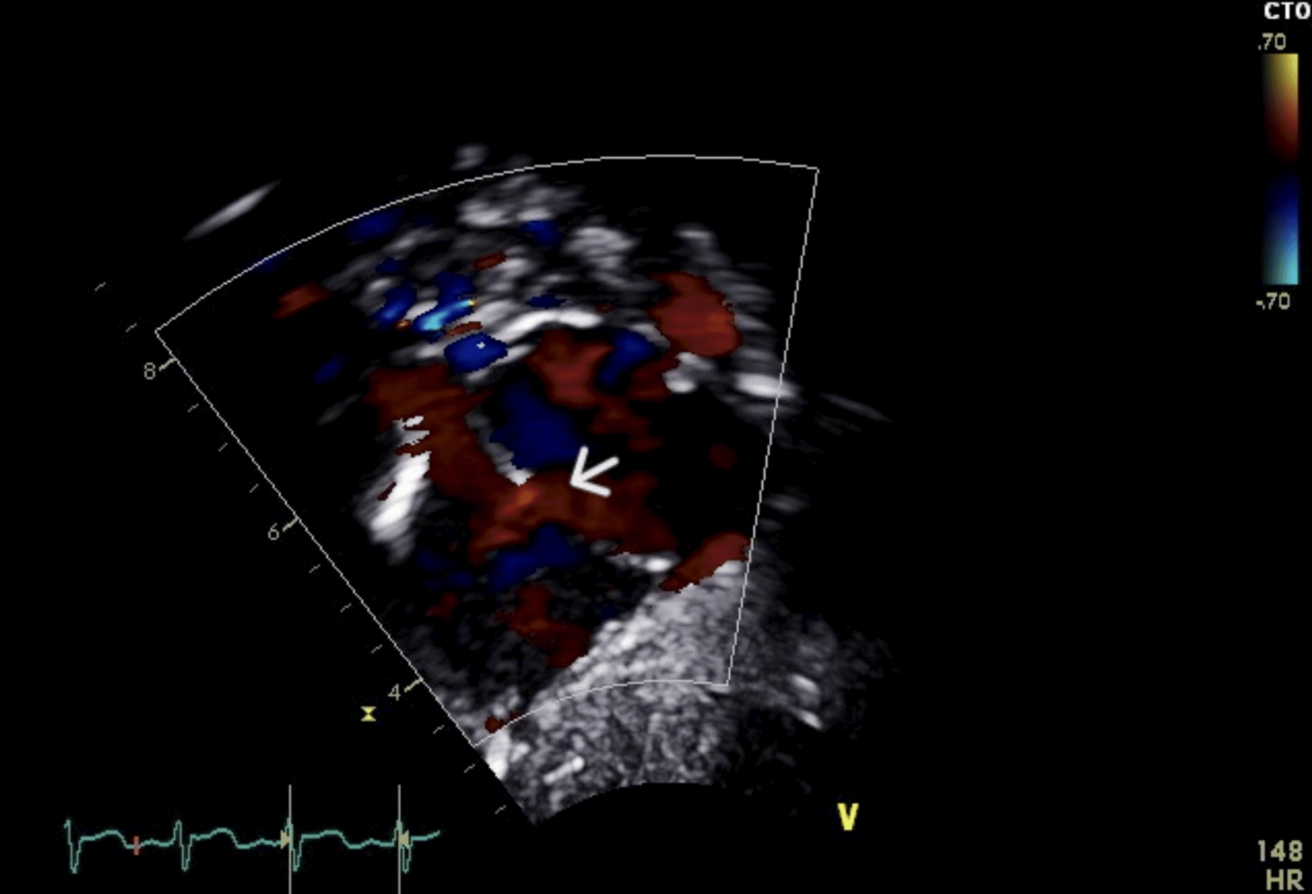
Doppler imaging of case one shows a small atrial septal defect with a left-to-right shunt.
